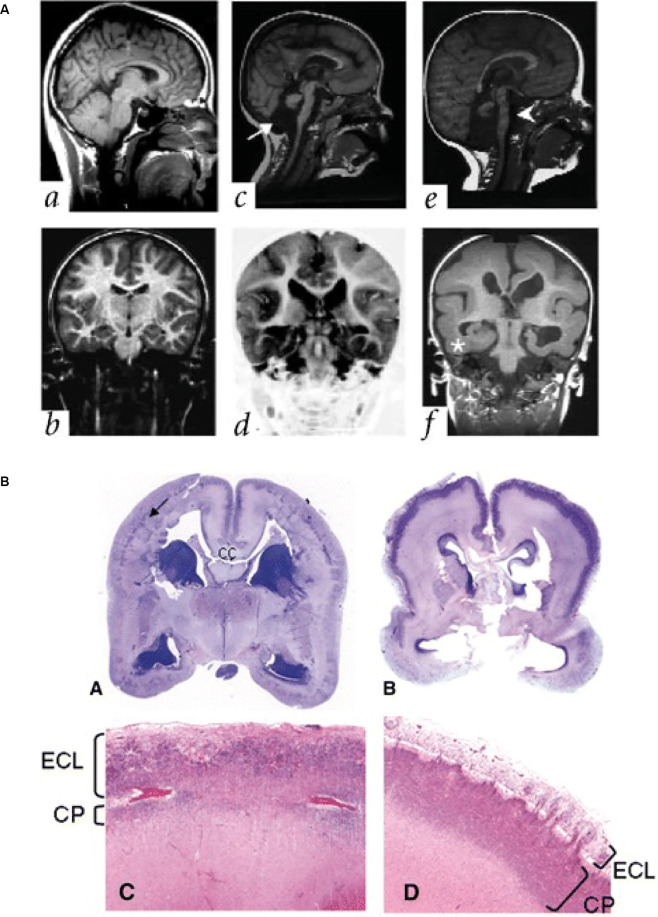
Lissencephaly in patients with mutations in ECM and ECM-associated genes. (A) Parasagittal (upper panels) and coronal (lower panels) MRI from patients with a normally developing brain (*a,b*), and two patients with lissencephaly associated with RELN mutations (*c,d*; *e,f*). Note the thickened cortex and reduced folding present. Adapted from Hong et al. (2000). (B) Images showing examples of cobblestone lissencephaly in a 19 pcw fetus with a POMT1 mutation (A,C, left panels) and 26 pcw fetus with a POMGNT1 mutation (B,D, right panels). The top panels show whole-mount sections of the brain. Arrow indicates impoverished cortical plate in the top left panel. The lower panels show higher magnification images of the cortical plate from these fetuses. Note the reduced size of the cortical plate (CP) and the thickened extracortical layer (ECL) in the lower left panel, but the thin ECL in the lower right panel. CC indicates the corpus callosum. Adapted from Devisme et al. (2012).
